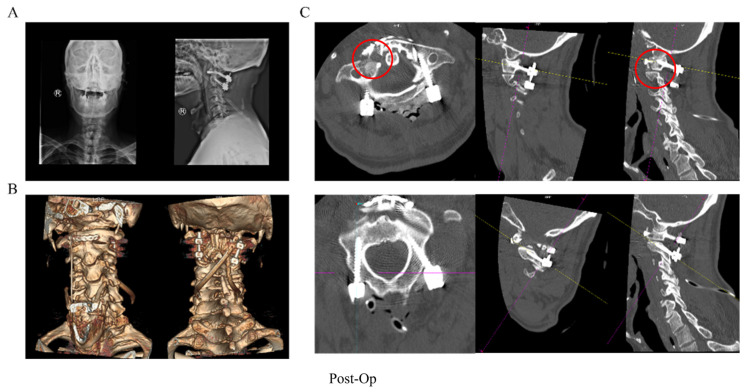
Postoperative imaging assessments: (A) Anteroposterior and lateral radiographs of the cervical spine. (B) Postoperative 3D reconstruction CT image. (C) Postoperative cervical CT; fracture sites and dislocated C1 lateral mass joints were reduced and fixed (red circle).
